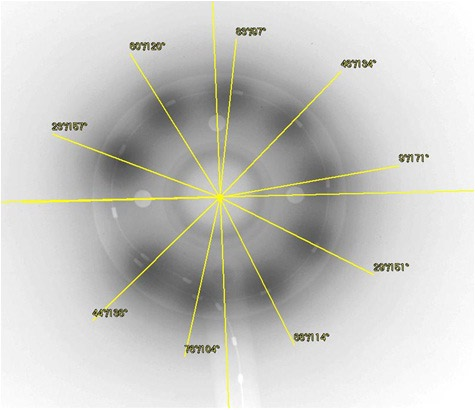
Conventional method: autoradiography and radiography on a single film for a 2 mm offset; film processed with K‐PACS software to measure the angles.
